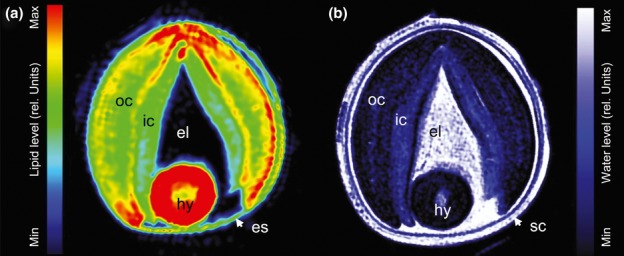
Distribution of (a) storage oils and (b) water in the developing oilseed rape (*Brassica napus*) seed (horizontal section). Visualization was achieved using non-invasive MRI. The concentration of both water and oil are colour-coded and given in relative units. Their three dimensional distribution is given in Movie S3. el, endospermal liquid; es, endosperm; hy, hypocotyl; ic, inner cotyledon; oc, outer cotyledon; sc, seed coat (testa).
